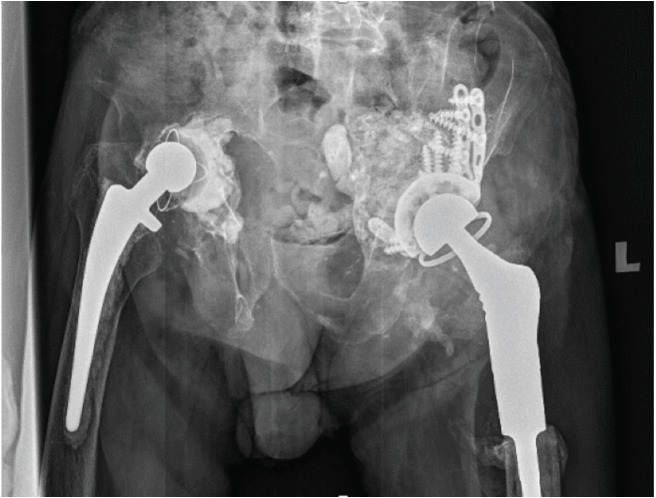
AP Pelvic radiograph showing a reconstructed left hip using a proximal femoral replacement and a cup cage with a cemented capture at two years follow-up.
